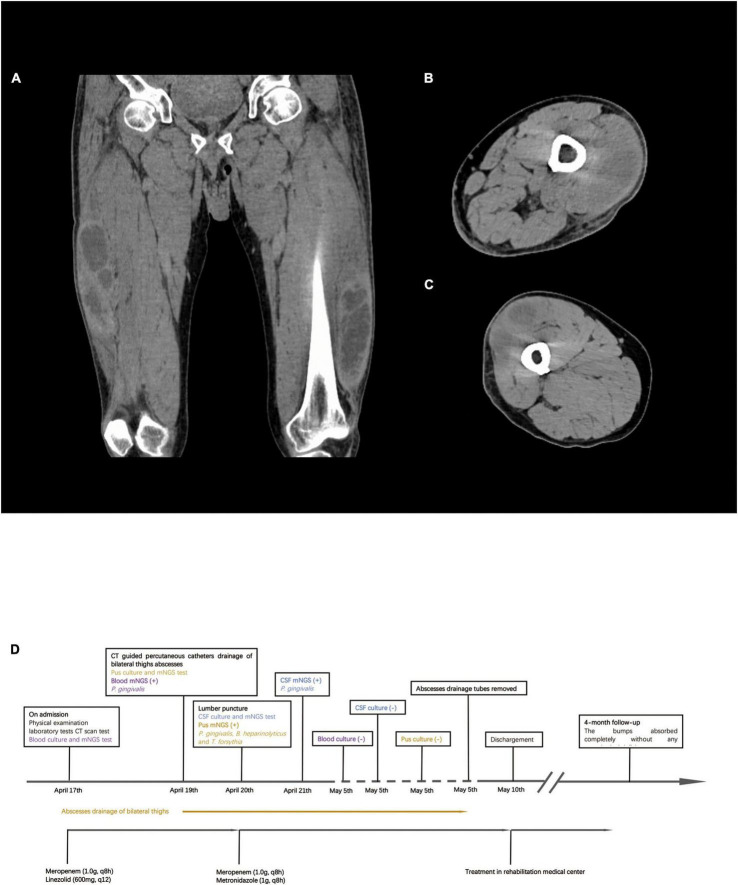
Computed tomography (CT) scan result of bilateral lower extremities and timeline of treatment. (A) CT scan shows multiple muscle abscesses in bilateral thighs. (B) Right thigh abscess. (C) Left thigh abscess. (D) Timeline of treatment.
